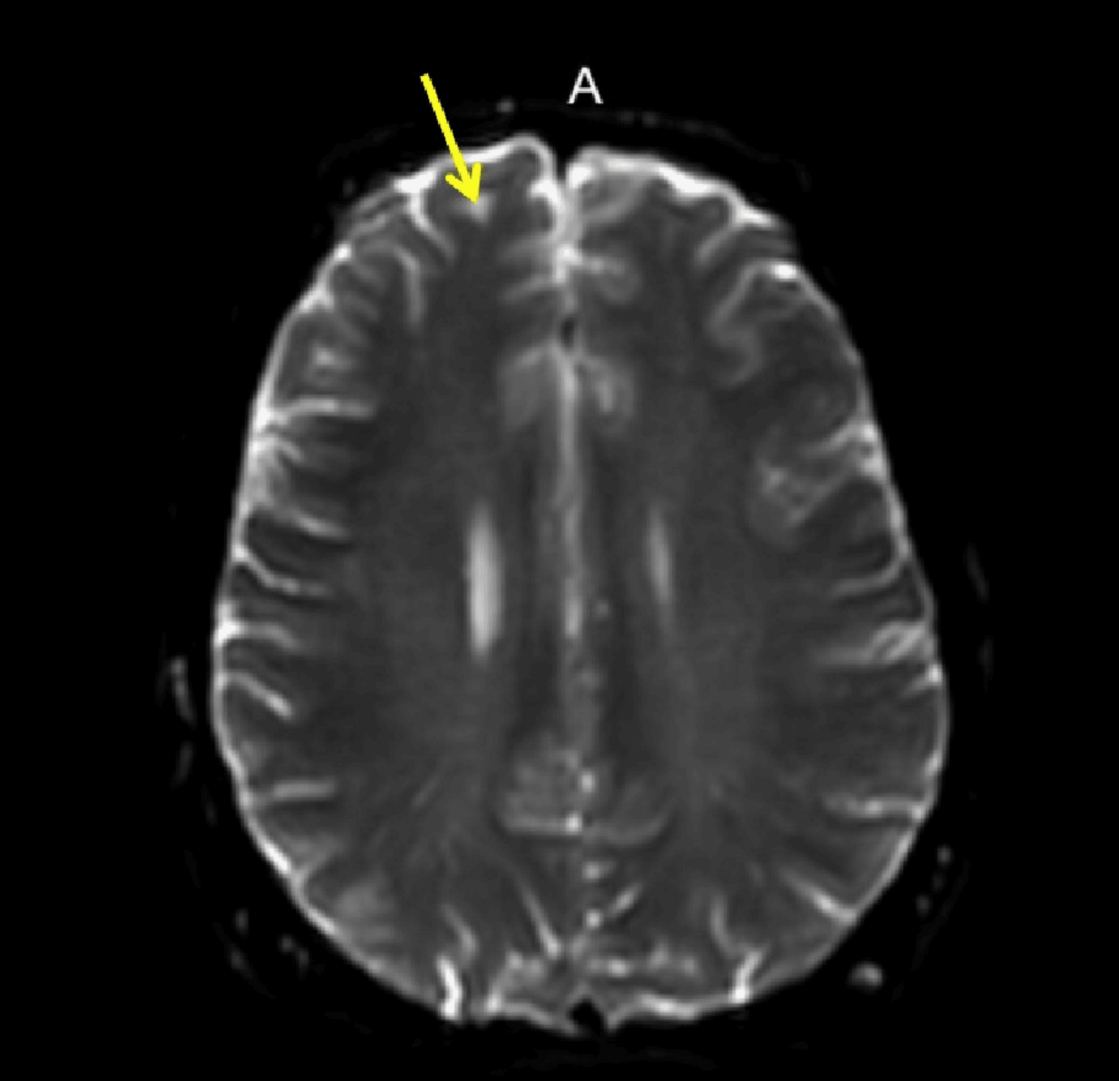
MRI axial view T2-weighted. There is a sub-centimeter focus of the hyperintense signal (arrow) within the white matter of the right frontal lobe (nonspecific) and could be sequela of migraine headaches or related to chronic small vessel ischemic change.
